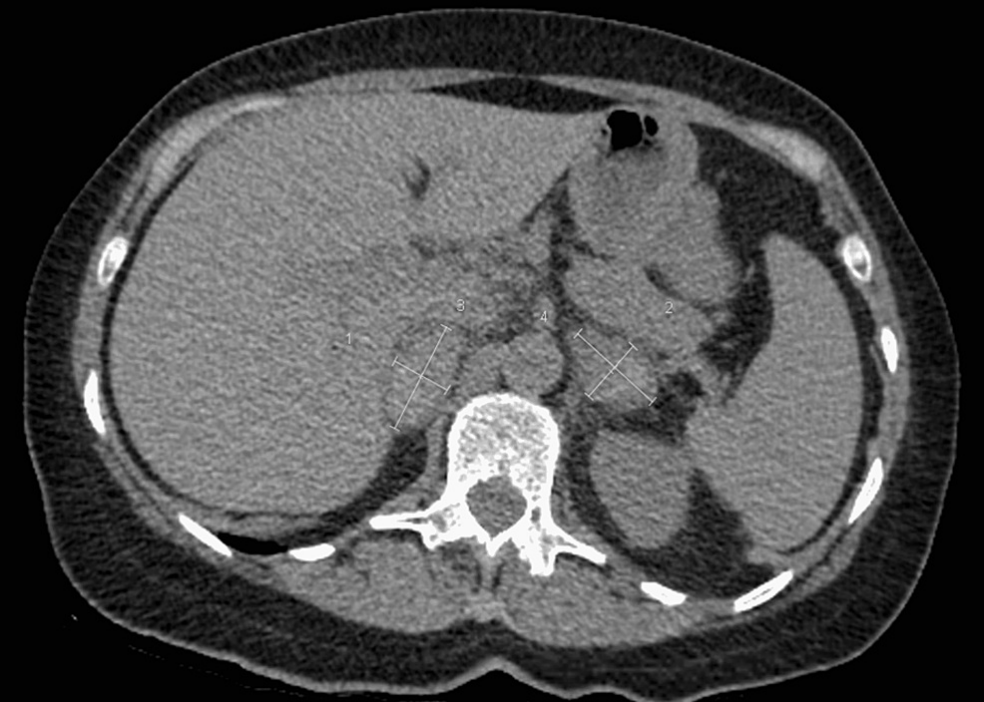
Axial view of CT scan and the bilateral adrenal hemorrhages
